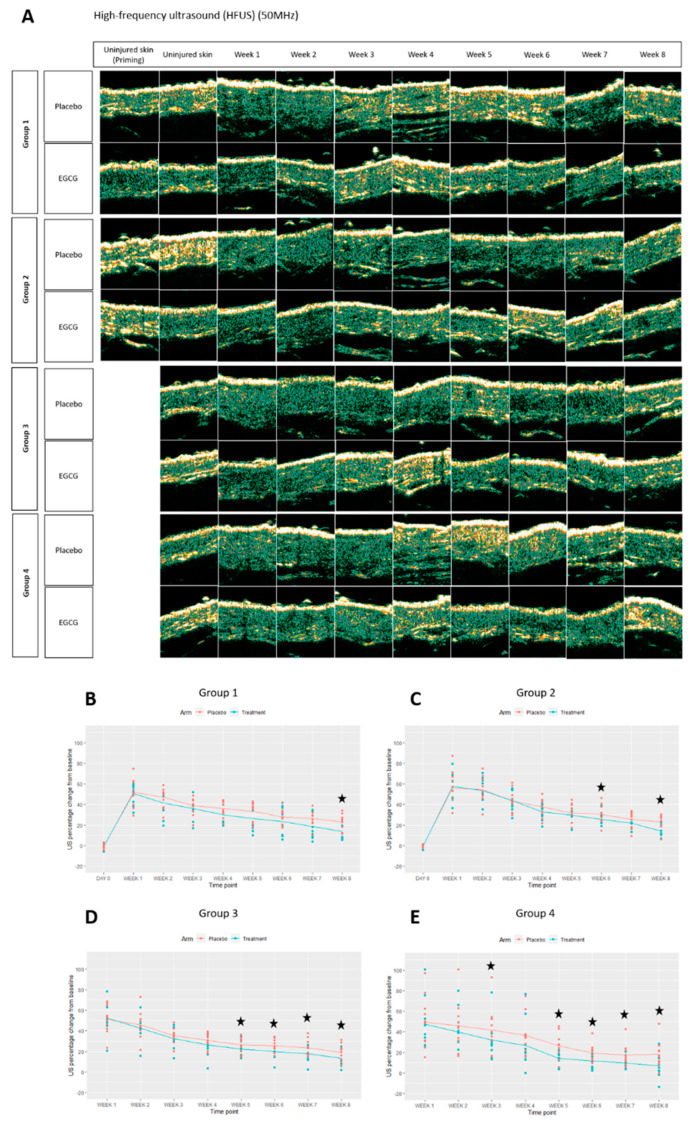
Skin thickness analysis (High frequency ultrasound (HFUS)) of topical epigallocatechin-3-gallate (EGCG) versus placebo. (A) Images of skin/scar thickness using high frequency ultrasound (HFUS). (B) Group 1 skin thickness HFUS. (C) Group 2 skin thickness HFUS. (D) Group 3 skin thickness HFUS. (E) Group 4 skin thickness HFUS. Significance: ★ *p* ≤ 0.01. Error bars: mean ± SD. Scale bars = 100 μm.
